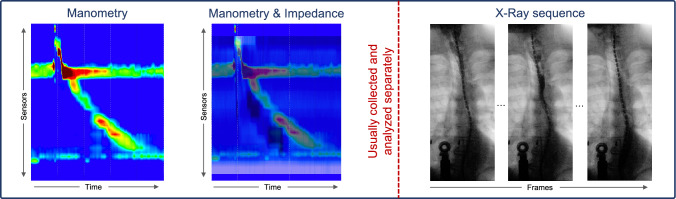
Examples of the three modalities manometry, impedance (overlaid on manometry), and X-ray images (videofluoroscopy) during a single swallowing act. Usually, the modalities are separately collected and analyzed, while in the present work we collect the modalities simultaneously and visualize the different modalities in a combined fashion
